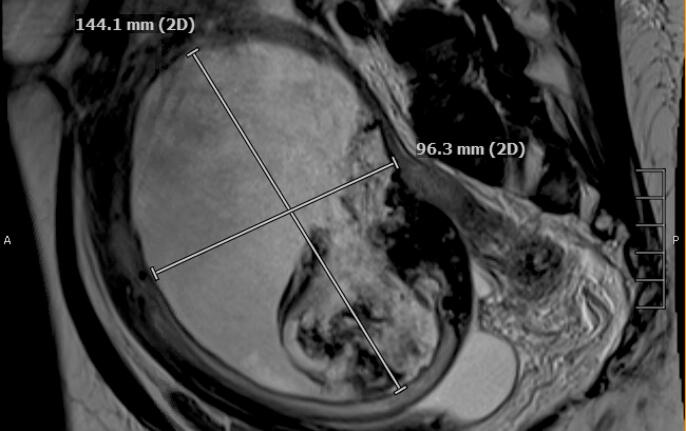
Findings of magnetic resonance imaging. A complex proteinaceous and calcified lesion is visible.
